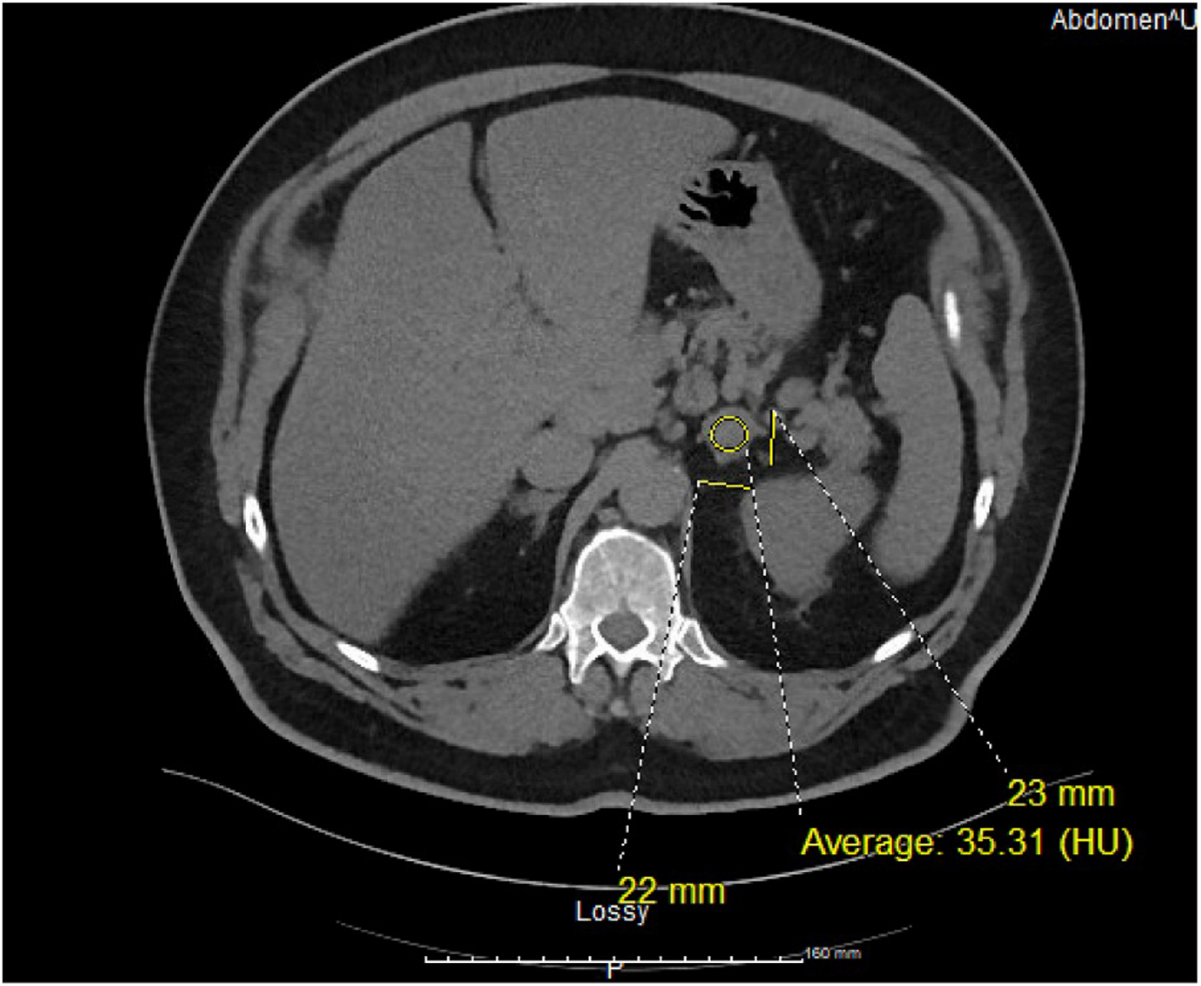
Noncontrast computed tomography (CT) abdomen and pelvis 3 years after initial cancer staging imaging showing *left* adrenal tumor sized 22 × 23 mm with 35.31 HU.
